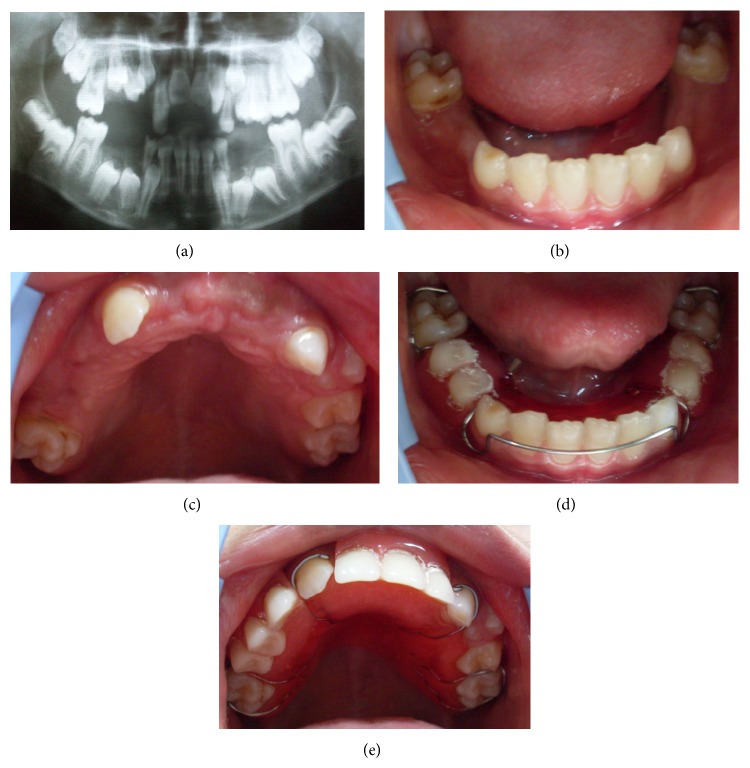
Intraoral and panoramic views 1 month after the extractions. (a) Postoperative panoramic radiograph. (b, c) Postoperative intraoral views of the patient. (d, e) Space maintainers were prepared for maxillary and mandibular regions.
